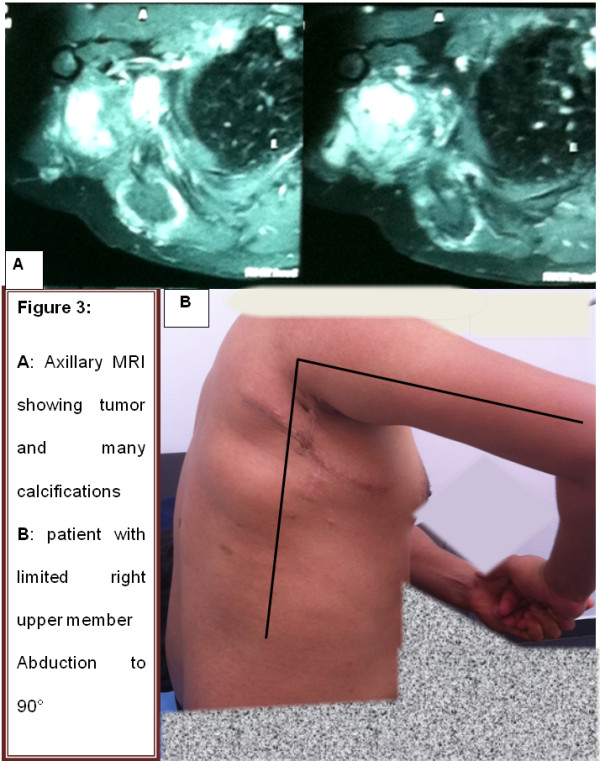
Last magnetic resonance imaging and patient status. Axillary magnetic resonance imaging (A) showing tumor and many intratumoral calcifications. (B) Patient with limited right upper member abduction to 90°.
